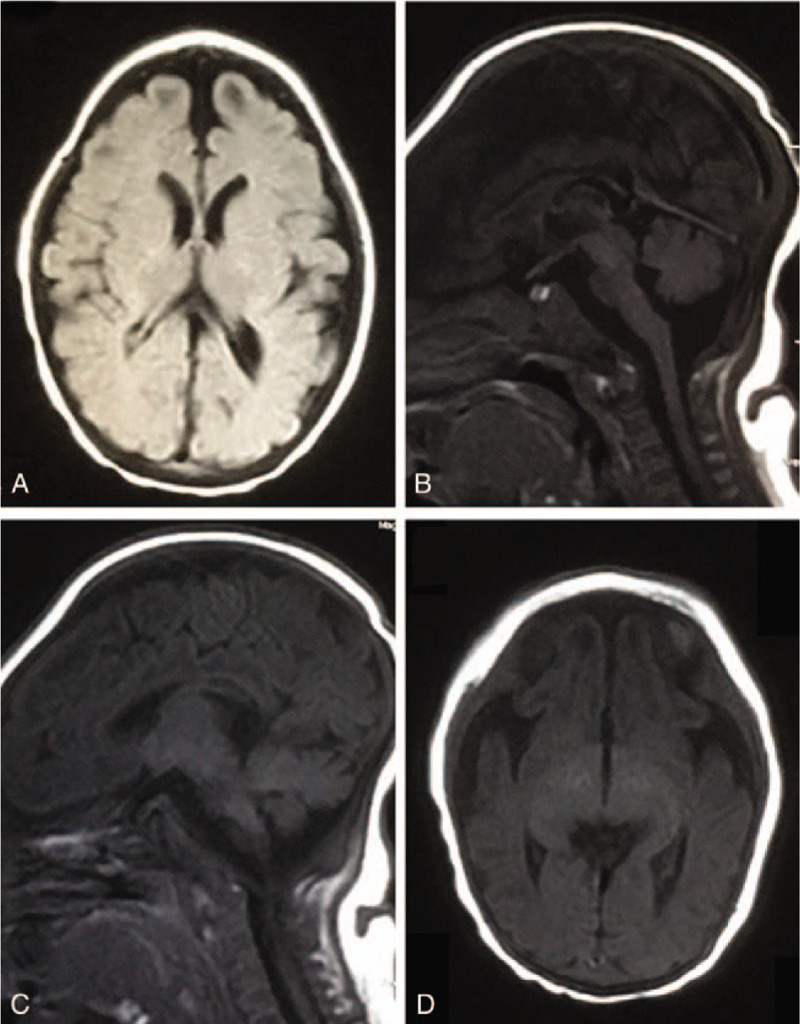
Brain magnetic resonance image (MRI) at 2 months after birth. Brain MRI showed dysplasia in the frontal lobes, corpus callosum, brainstem, and cerebellum along with widening of the cerebral sulci and gyrus.
